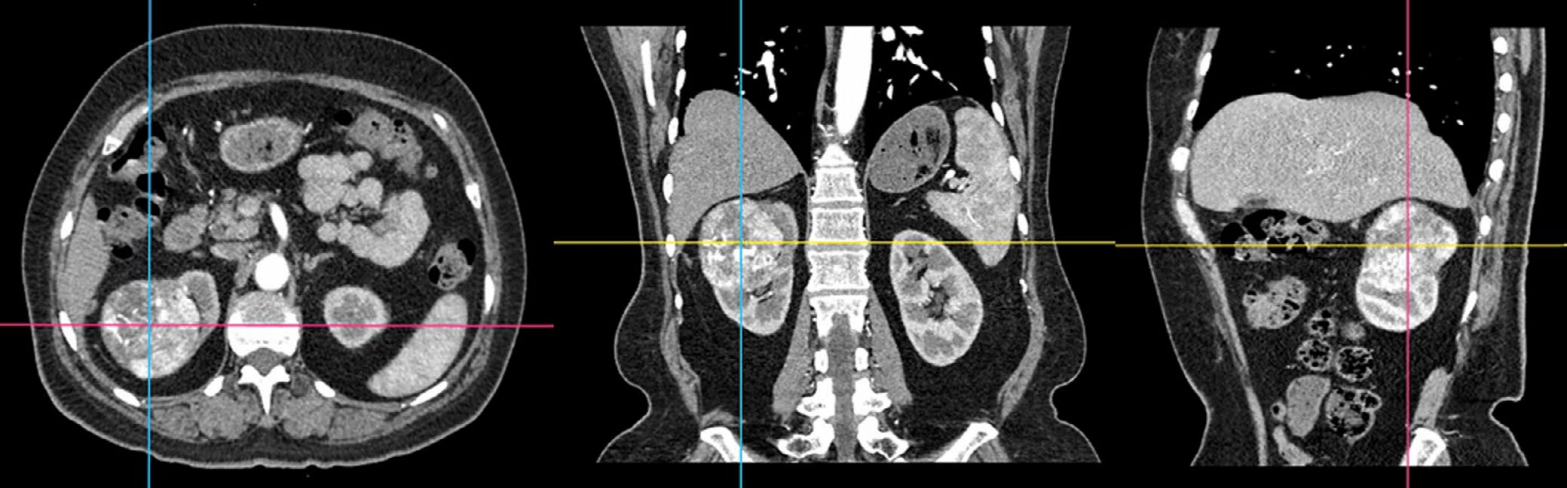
CT DICOM data acquisition and multiplanar reconstruction (MPR)
